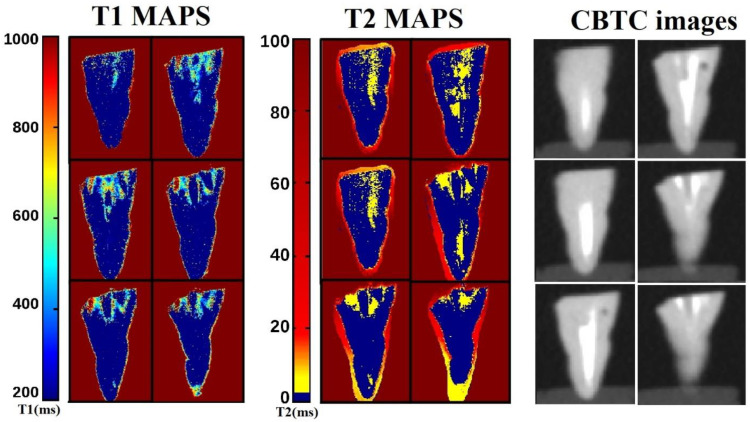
T1, T2 maps and CBCT images obtained from the same tooth slices showing the different and complementary image contrast assessed by mMRI maps and CBCT images. Water is present inside the canal, both in the coronal, middle and apical thirds, showing an incomplete three-dimensional filling and consequently fluid leakage. By considering the T1 and T2 values, in the last apical 3 mm, it is possible to evidence the presence of some inorganic tissue and water (light blue and yellow areas).
